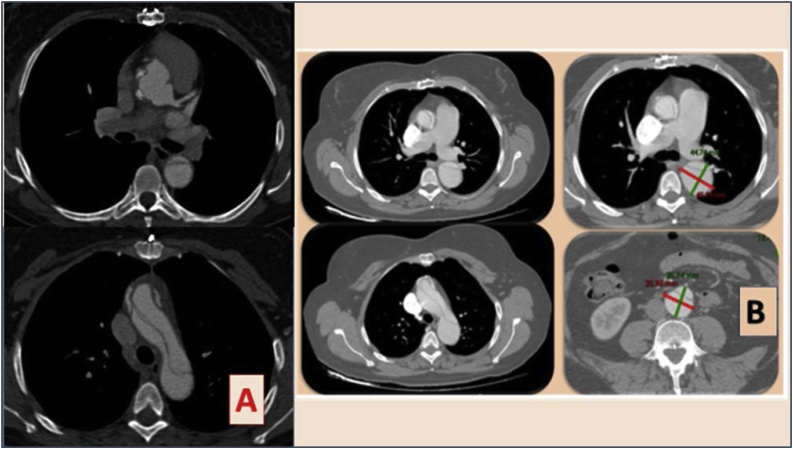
A Pre-Operatory CT scan- aortic valve replacement. B Follow up CT scan, 10 years from aortic dissection.
